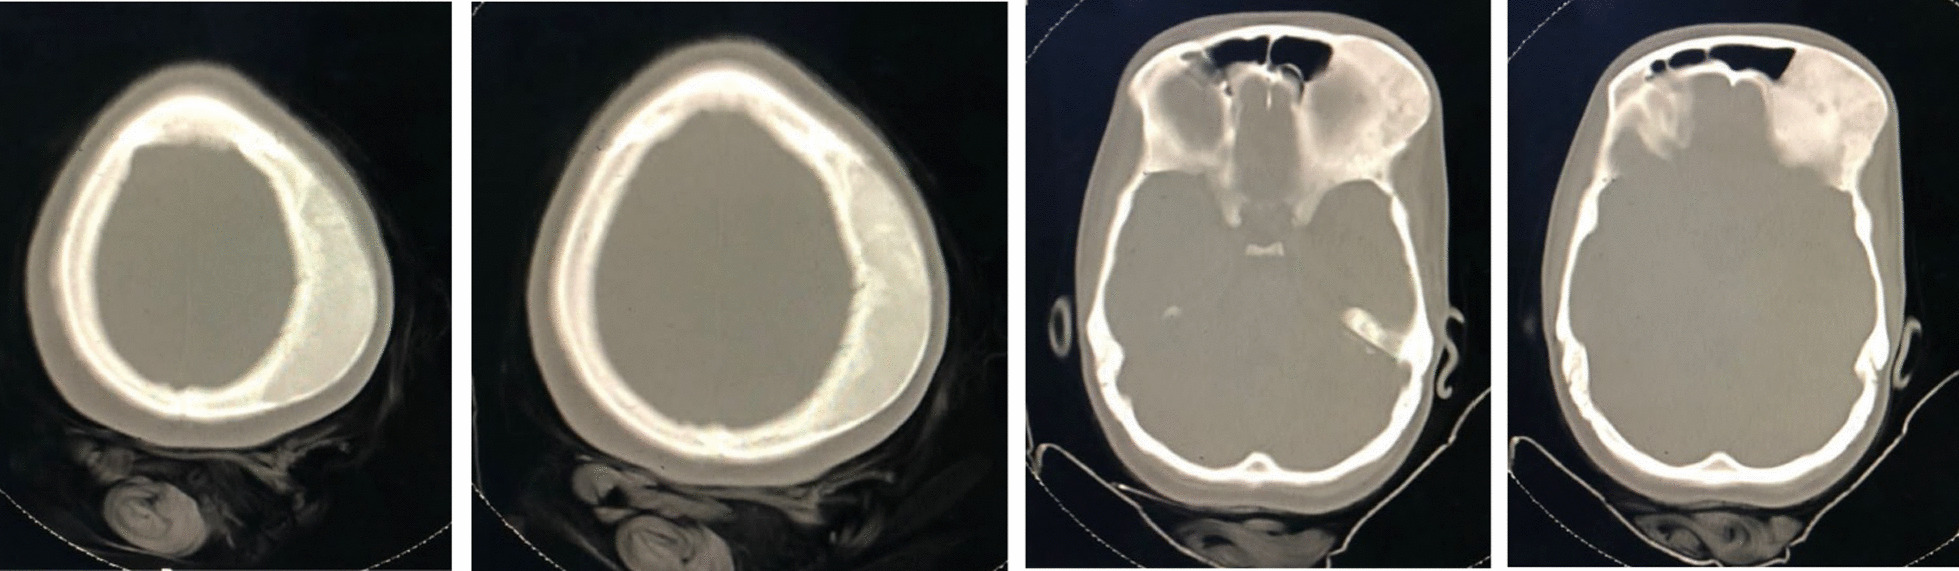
Head CT scan showing thickness in the space between the two bone plates in the left frontoparietal region, which reached the orbital roof without cortical destruction or periosteum reaction; the tumor size was 5 cm × 5 cm
